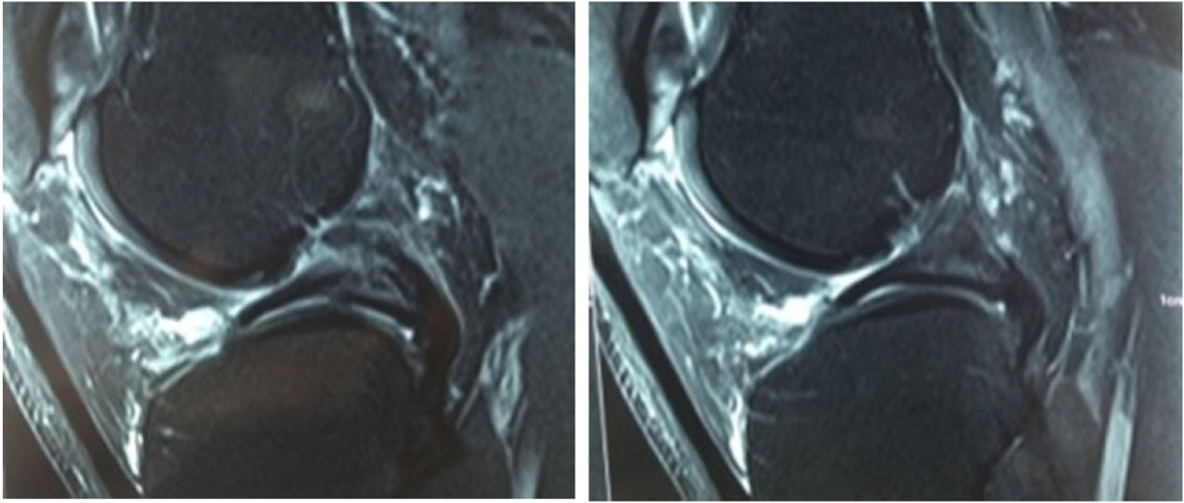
Magnetic resonance imaging in sagittal T2 demonstrating the meniscal variant of posterior junction and insertion to the anterior cruciate ligament
